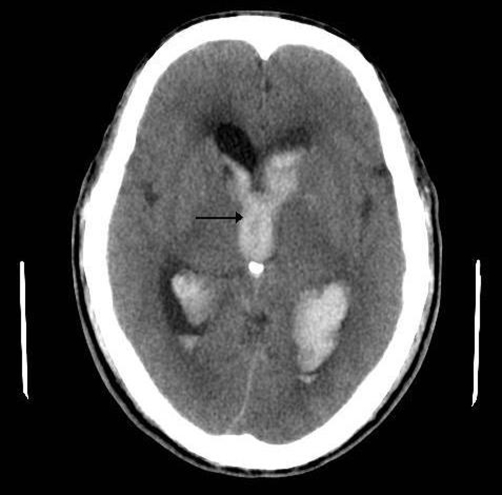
CT head; CT scan of the patient’s head demonstrated high attenuation areas in the ventricles, indicating intraventricular bleeding (arrow).
